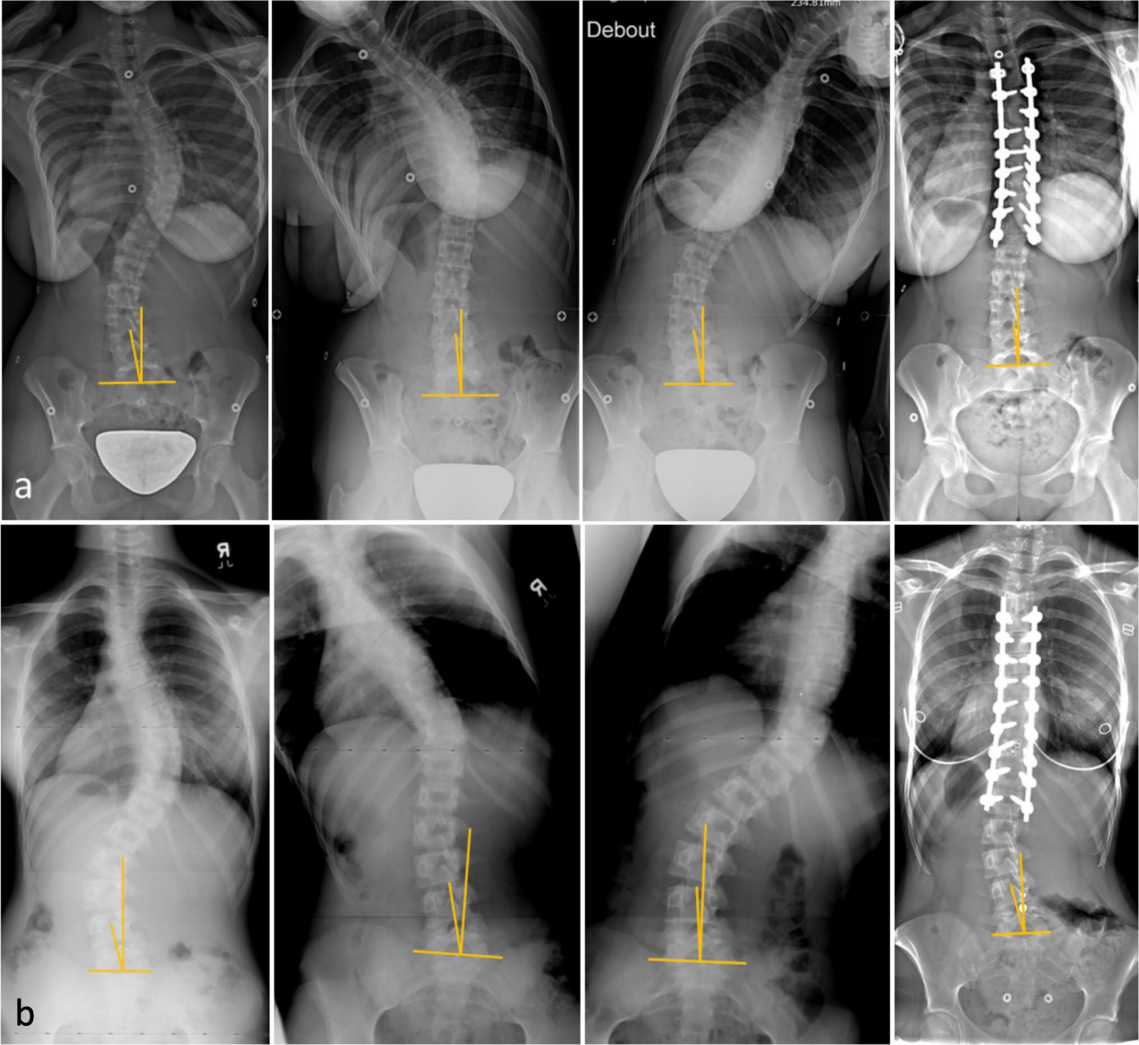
a Preoperative and postoperative PA radiographs of ‘flexible’ LSTOA, with an initial LSTOA of 8.5 that measured 2.2 with ipsilateral bending and 3.2 postoperatively. b Preoperative and postoperative PA radiographs of ‘ridged’ LSTOA, with an initial LSTOA of 15.5 that measured 15.8 with ipsilateral bending and 15.6 postoperatively
